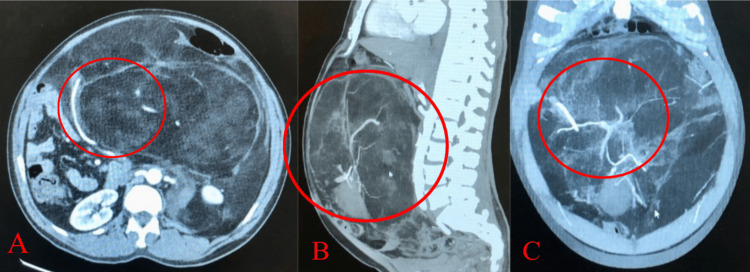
Computed tomography angiography with intravenous contrast depicted a large lipomatous abdominal mass extending from the upper abdomen to the pelvis and measured approximately 32 cm, in the axial (A), sagittal (B), and coronal planes (C). Internal septations were noted. Arterial supply appeared to be originating from the inferior mesenteric artery and a secondary branch was noted on the infrarenal abdominal aorta (red circles). Venous drainage appeared to be via the inferior mesenteric vein.
